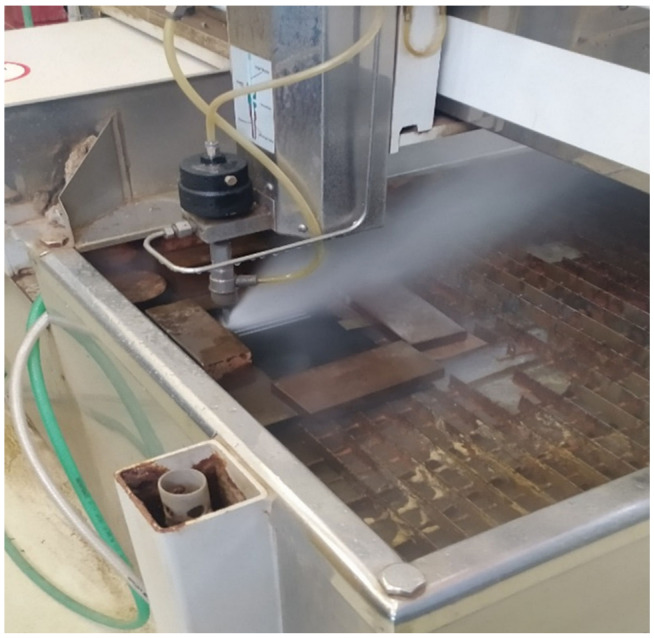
AWAC CNC waterjet cutting machine AWJ CT 0806: waterjet cutting process.
